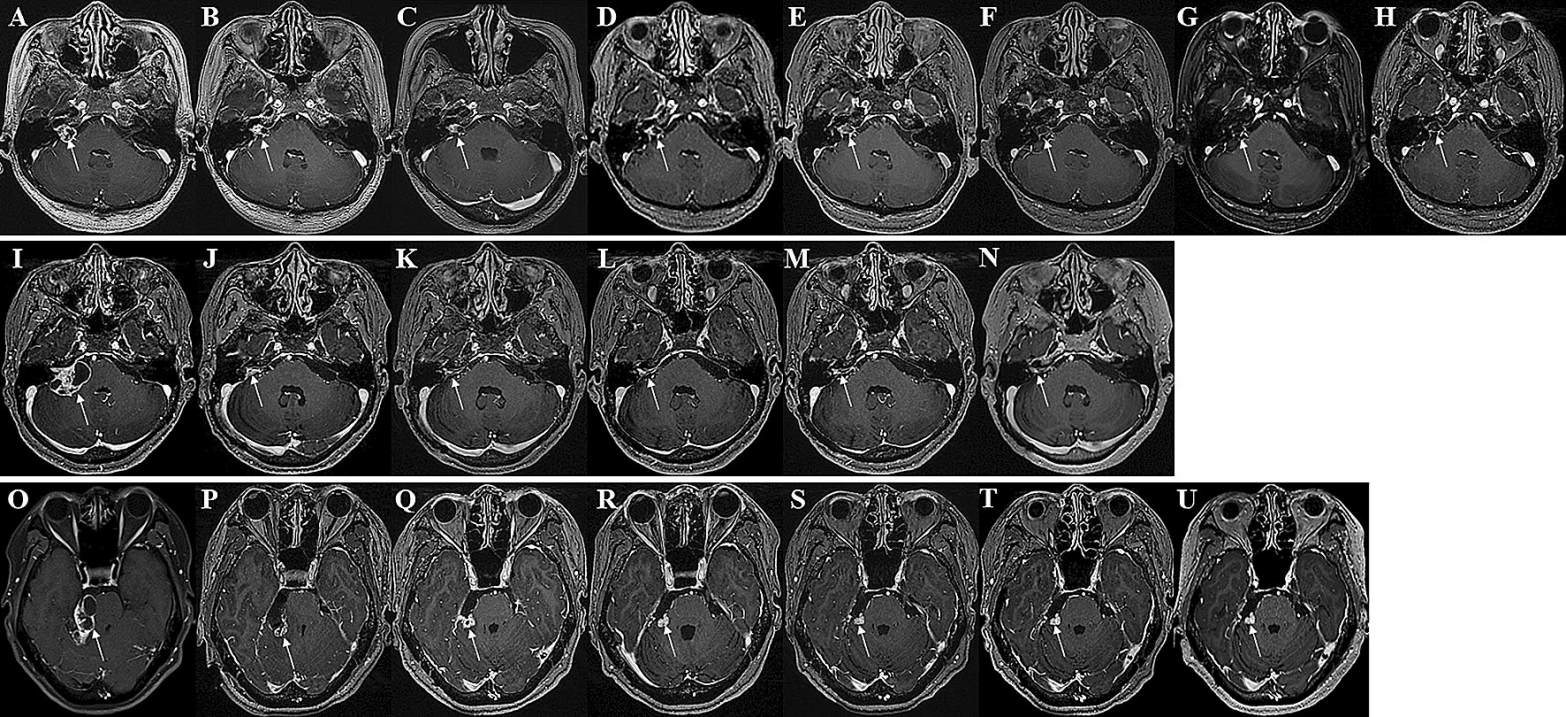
Preoperative (A,I,O), immediate (B,J,P), 1- (C,K,Q), 2- (D,L,R), 3- (E,M,S), 5- (F,N,T), 7- (G,U), and 10-year (H) postoperative gadolinium enhanced T1-weighted axial MR images of patients with residual tumor (white arrows) following incomplete resection. (A–H) The residual tumor in IAC of a patient with partial resection (85.2% extent of resection) gradually regressed from an immediate postoperative tumor volume of 1.19 cm^3^ (B) to 0.01 cm^3^ (H) over a follow-up of 147 months. The patient had a normal facial nerve function at last follow-up. (I–N) The residual tumor in IAC of a patient with subtotal resection (93% extent of resection) gradually regressed from an immediate postoperative tumor volume of 0.62 cm^3^ (J) to 0.2 cm^3^ (N) over a follow-up of 62 months. The patient had a normal facial nerve function at last follow-up. (O–U) The residual tumor at the brain stem of a patient with near-total resection (96.2% extent of resection) gradually regressed from an immediate postoperative tumor volume of 0.67 cm^3^ (P) to 0.38 cm^3^ (U) over a follow-up of 84 months. The patient had a facial nerve function of HB grade II at last follow-up.
